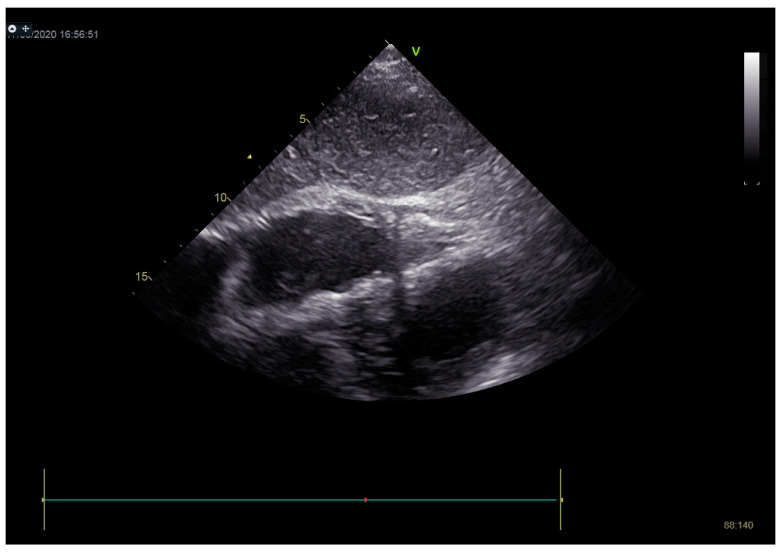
On echocardiography after pericardiocentesis, pericardial effusion was not registered.
